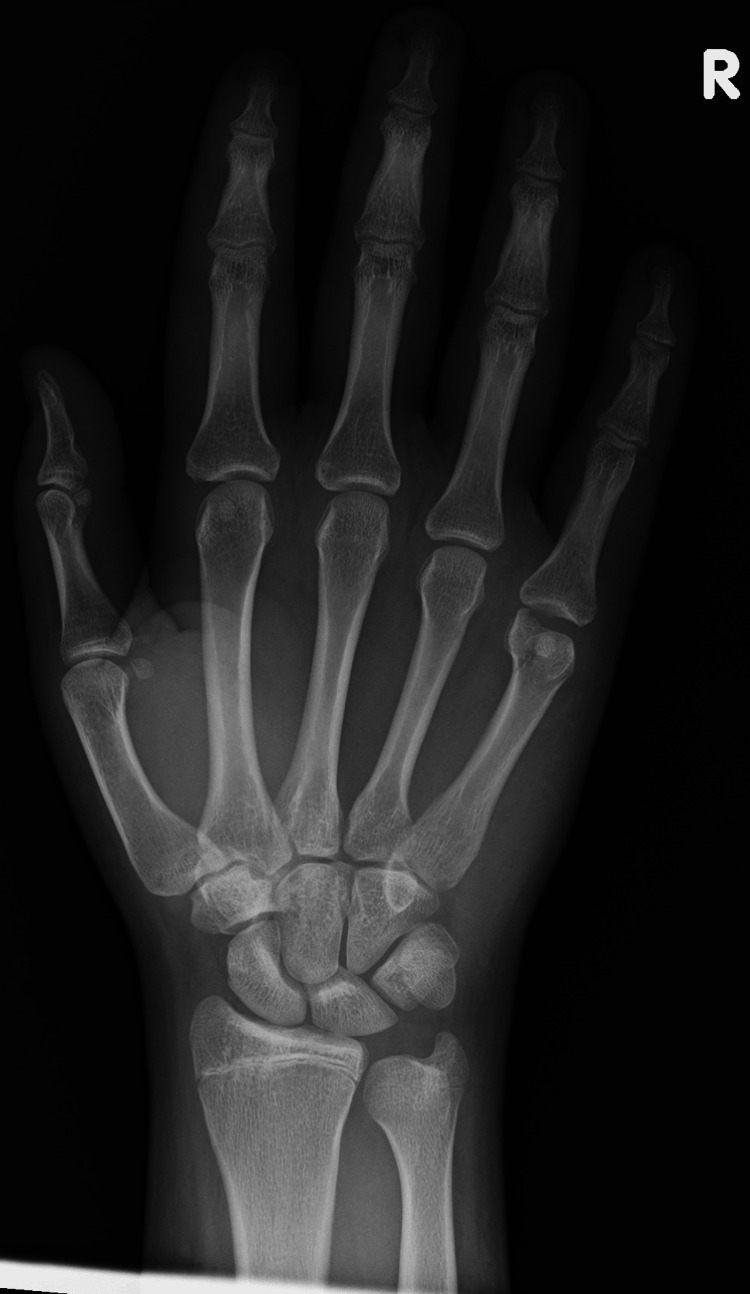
The patient's posteroanterior radiograph taken on presentation to the emergency department.
